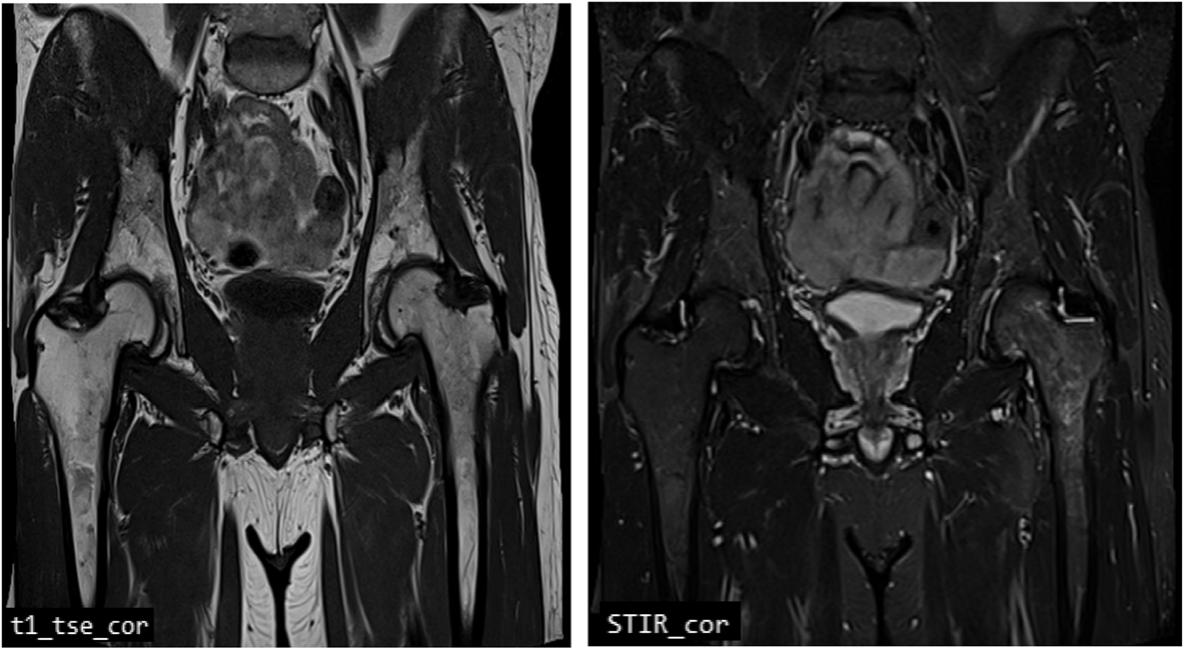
Left hip MRI showing a significant reduction of bone edema at proximal femur
